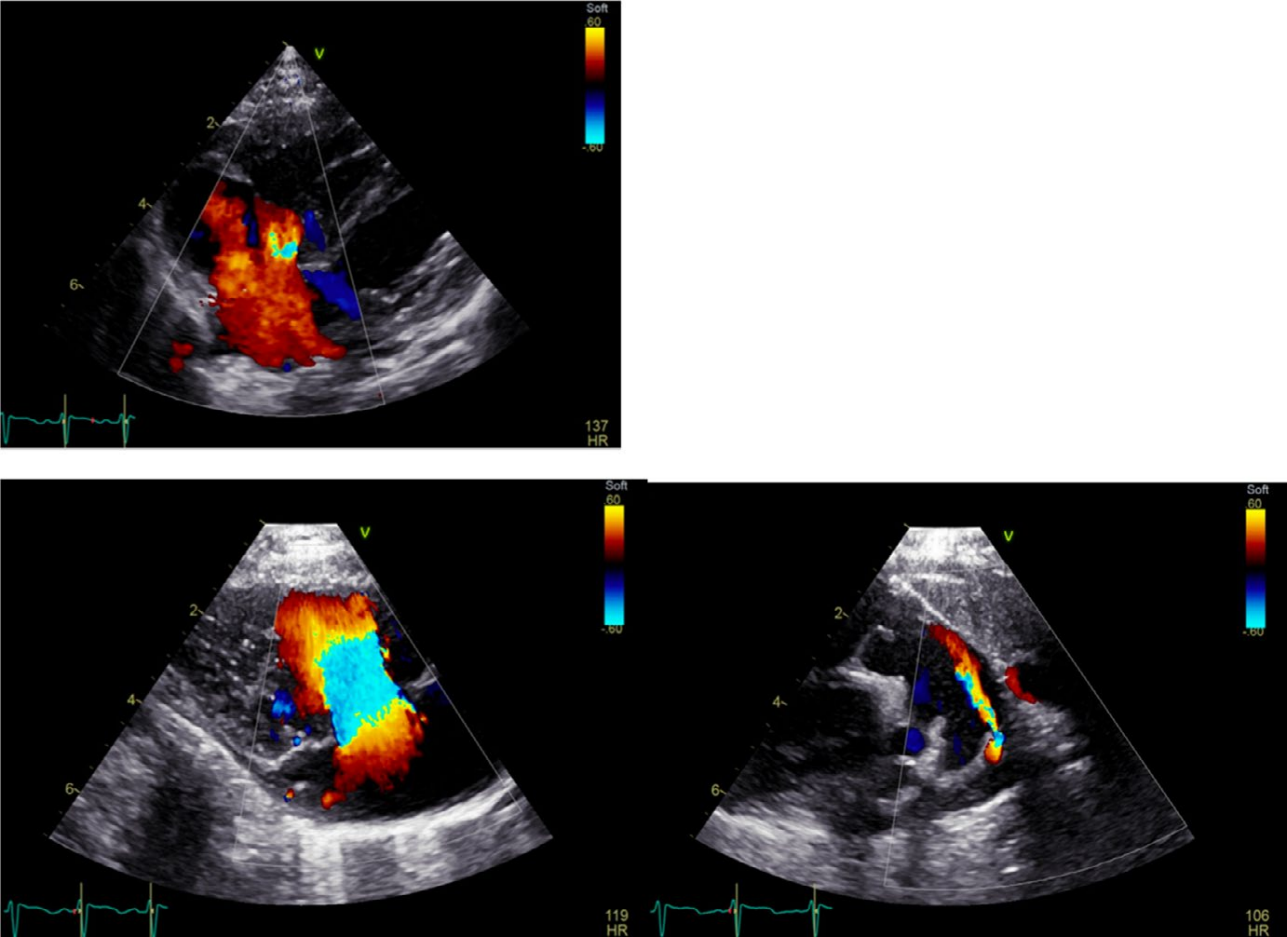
Preoperative echocardiography showing a prominent atrial septal defect jet and a patent ductus arteriosus
